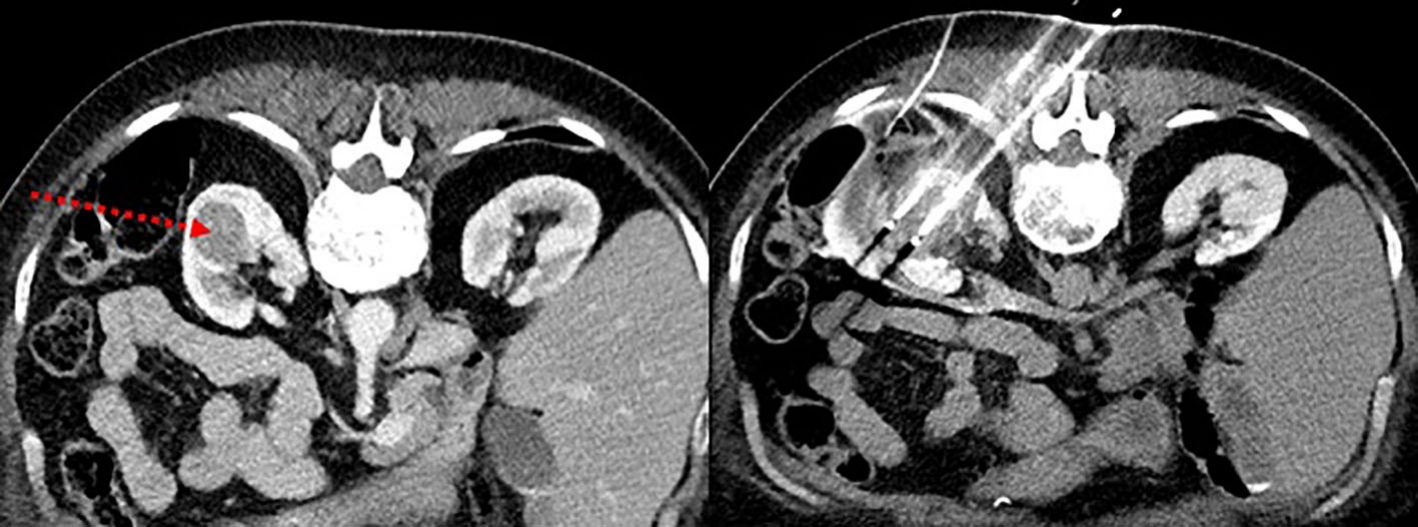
Pre- and per-procedure images of renal cryoablation. (Left picture) CT scan showing a left renal mass of 42 mm to treat (see arrow). (Right picture) Visualization of the inserted cryoprobes and the ice ball clearly circumscribing the tumor lesion.
